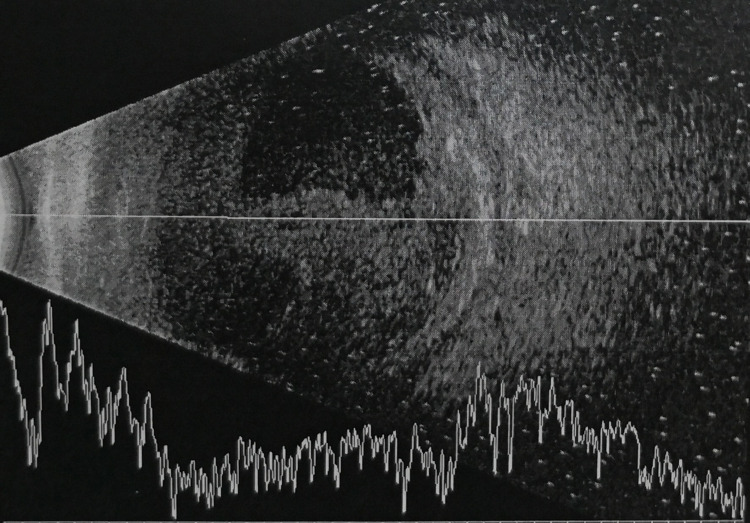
B-scan ultrasonography showed vitreous opacity and multiple loculations in the posterior chamber
